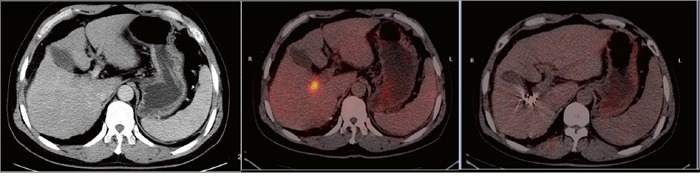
A 45-year-old man with hepatocellular carcinoma complicated with portal vein tumor thrombus in the right branch Enhanced computed tomography showed the thrombus originated from the right branch of the portal vein. Positron emission tomography-computed tomography demonstrated increased fluorodeoxyglucose uptake in the right branch of the portal vein. Positron emission tomography-computed tomography demonstrated no fluorodeoxyglucose uptake in the portal vein tumor thrombus two months after seed implantation.
